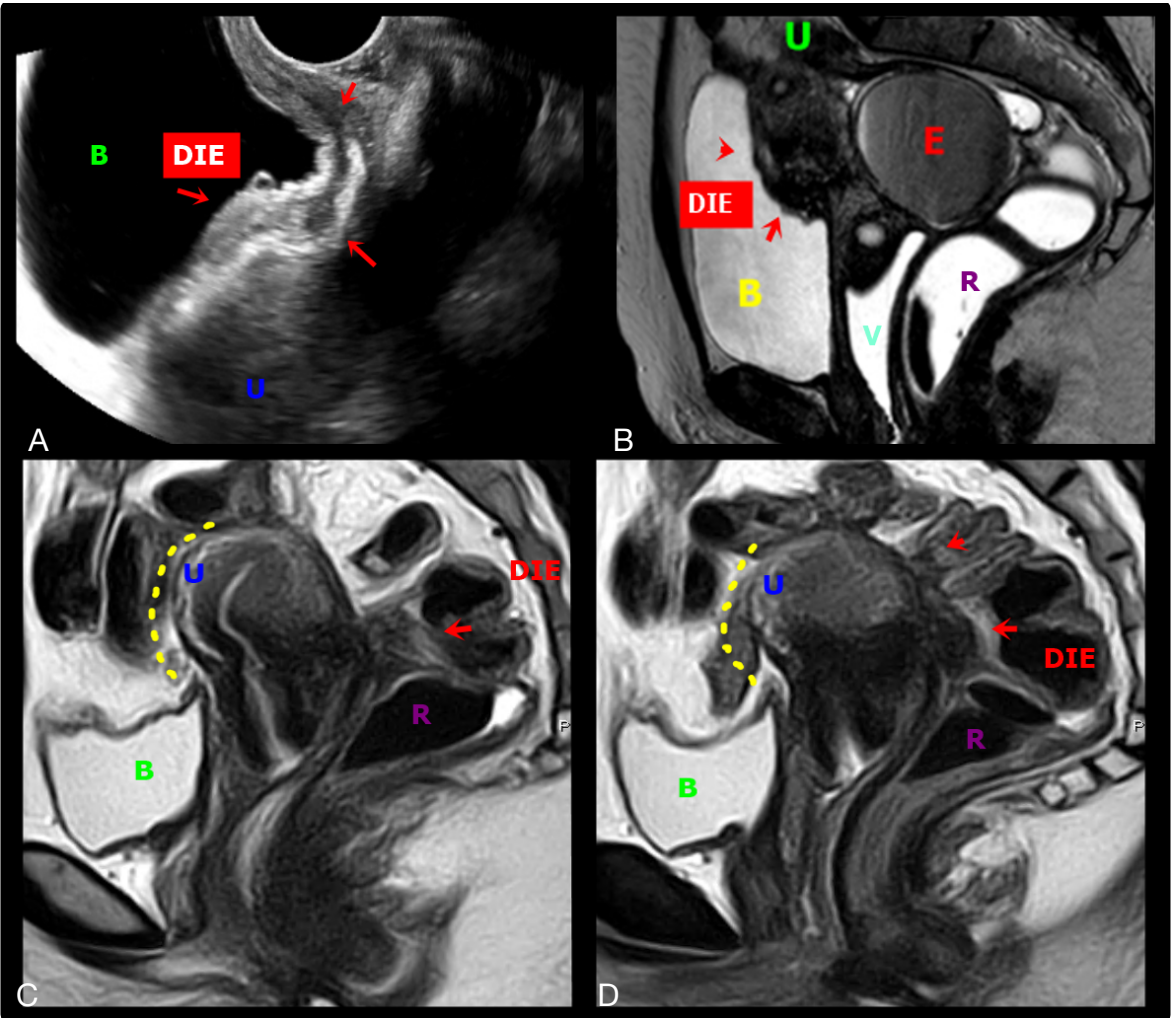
A & B. Transvaginal ultrasound and axial T2-weighted MR images show deep endometriosis (DIE, red arrows) obliteration of the peritoneum of the vesico-uterine space with involvement of the posterosuperior bladder wall. The lesion infiltrates the peritoneum of the vesicouterine space, causing the obliteration of the fatty planes between adjacent structures. B: Bladder, U: Uterus, R: Rectum, E: Endometrioma, V: Vagina; C & D. Sagittal T2W MR images show a deep endometriosis (DIE) plaque in the retrocervical space with bowel involvement, causing obliteration of the posterior cul-de-sac with loss of fat planes between adjacent structures and forced retroflexion of the uterine fundus (yellow interrupted line). B: Bladder, U: Uterus, R: Rectum, E: Endometrioma
